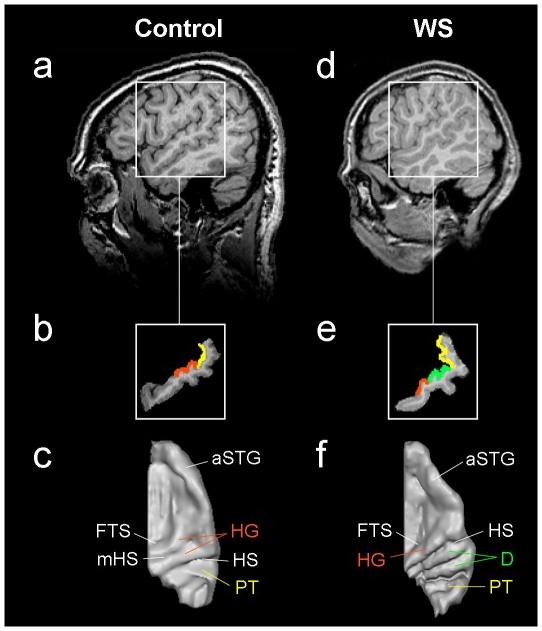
Anatomical landmarks of the auditory cortex. Auditory cortex (AC) of one control person (a–c) and one WS subject (d–e). Sagittal MR image at TAL x = 50 (a,d: left side of the image is the anterior part of the brain). Segmented STG (b,e) including Heschl's gyrus (HG; marked orange), planum temporale (PT; marked yellow) and two posterior duplications of HG in the WS subject (D; marked green). Three-dimensional surface reconstruction of right AC (c,f) reveals anatomical features and individual peculiarities such as D (f) or medial Heschl's sulcus (mHS; c). FTS =  first transverse sulcus; HS =  Heschl's sulcus; aSTG =  anterior superior temporal gyrus.
